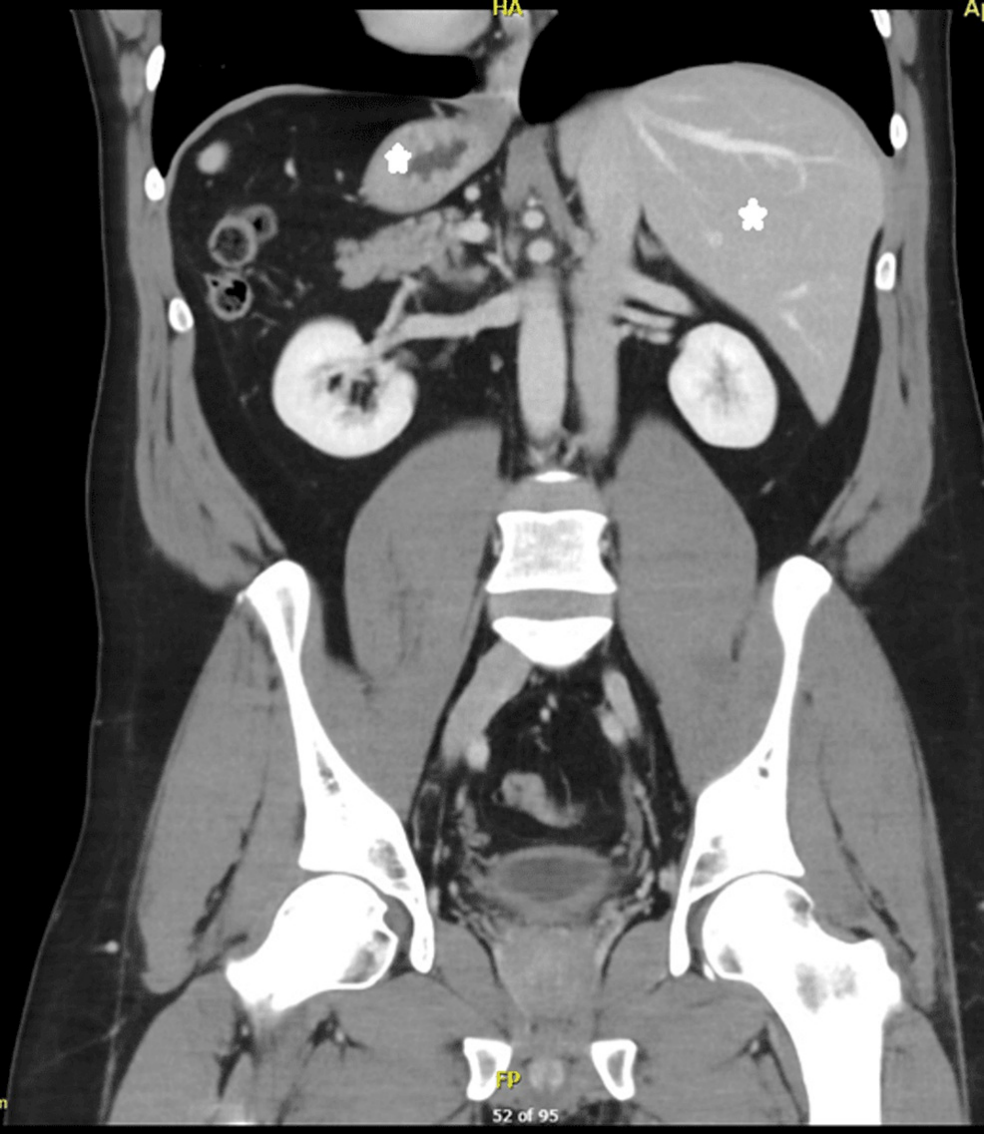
CT of the abdomen with angiogram showing intra-abdominal organs located in reverse, including the liver and stomach, representing SIT The star on the right side denotes the stomach, while the left one denotes the liver. CT: computed tomography; SIT: situs inversus totalis
